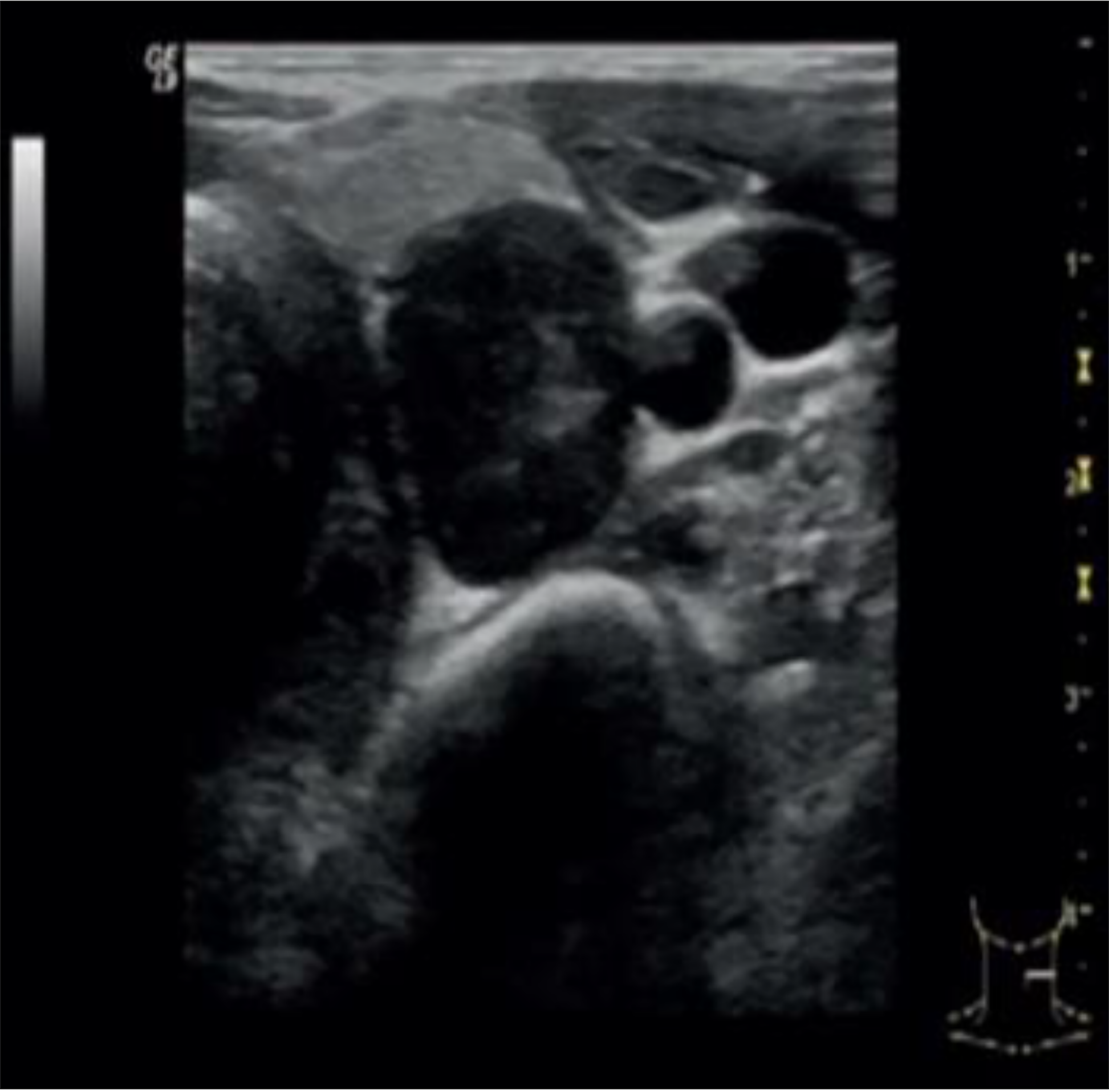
Neck ultrasound showed a 3.6 cm round hypoechoic nodule posterior to the left inferior thyroid lobe with undefined margins; no signs of infiltration of local tissues were detected.
